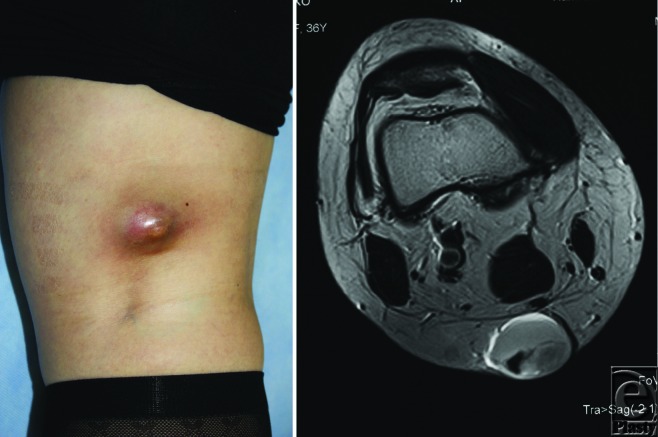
The tumor in the right popliteal fossa (naked eye appearance and MRI).
